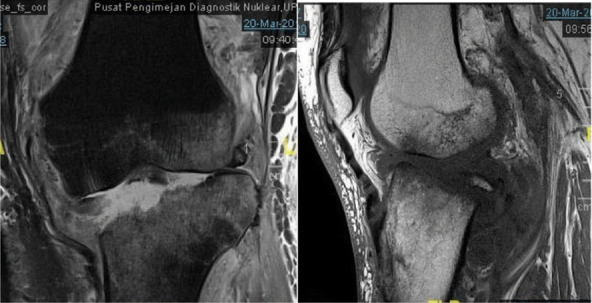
MRI scan of the left knee showing marked destruction of the tibial plateau in the coronal view. The sagittal view shows femoral condyle is also involved.Involvement of both distal and proximal surfaces of the joint strongly suggest infective pathology.
